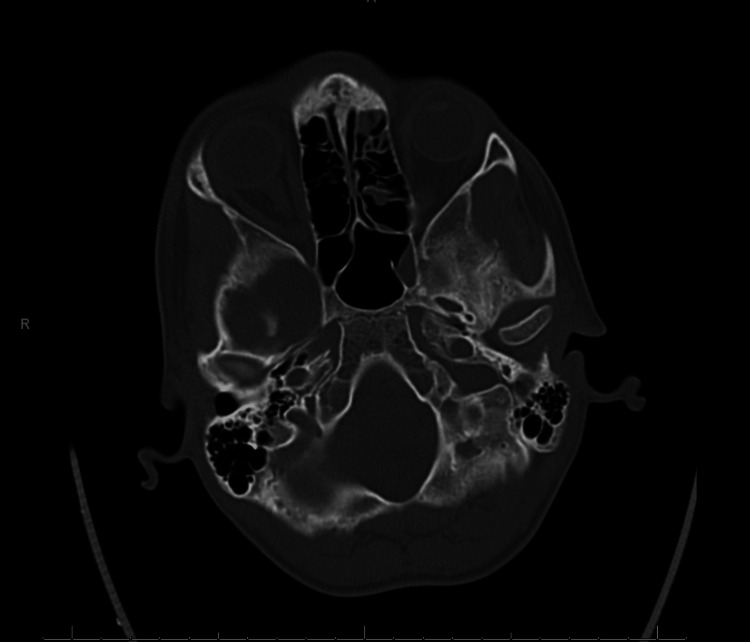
Non-contrast axial CT images of the head and temporal bones demonstrating no acute intracranial abnormality and normal-appearing internal auditory canals in this adolescent with sudden left-sided hearing loss and vertigo.
